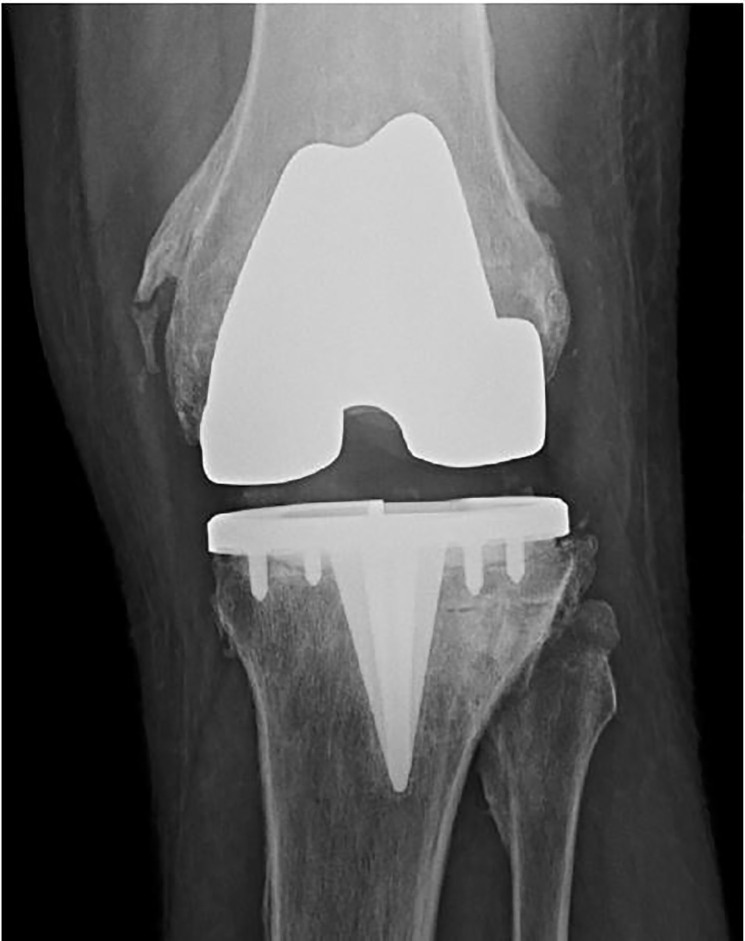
Anteroposterior radiograph of the left knee showing no evident hardware failure in a patient with history of knee replacement who presented with pain and decreased range of motion.
